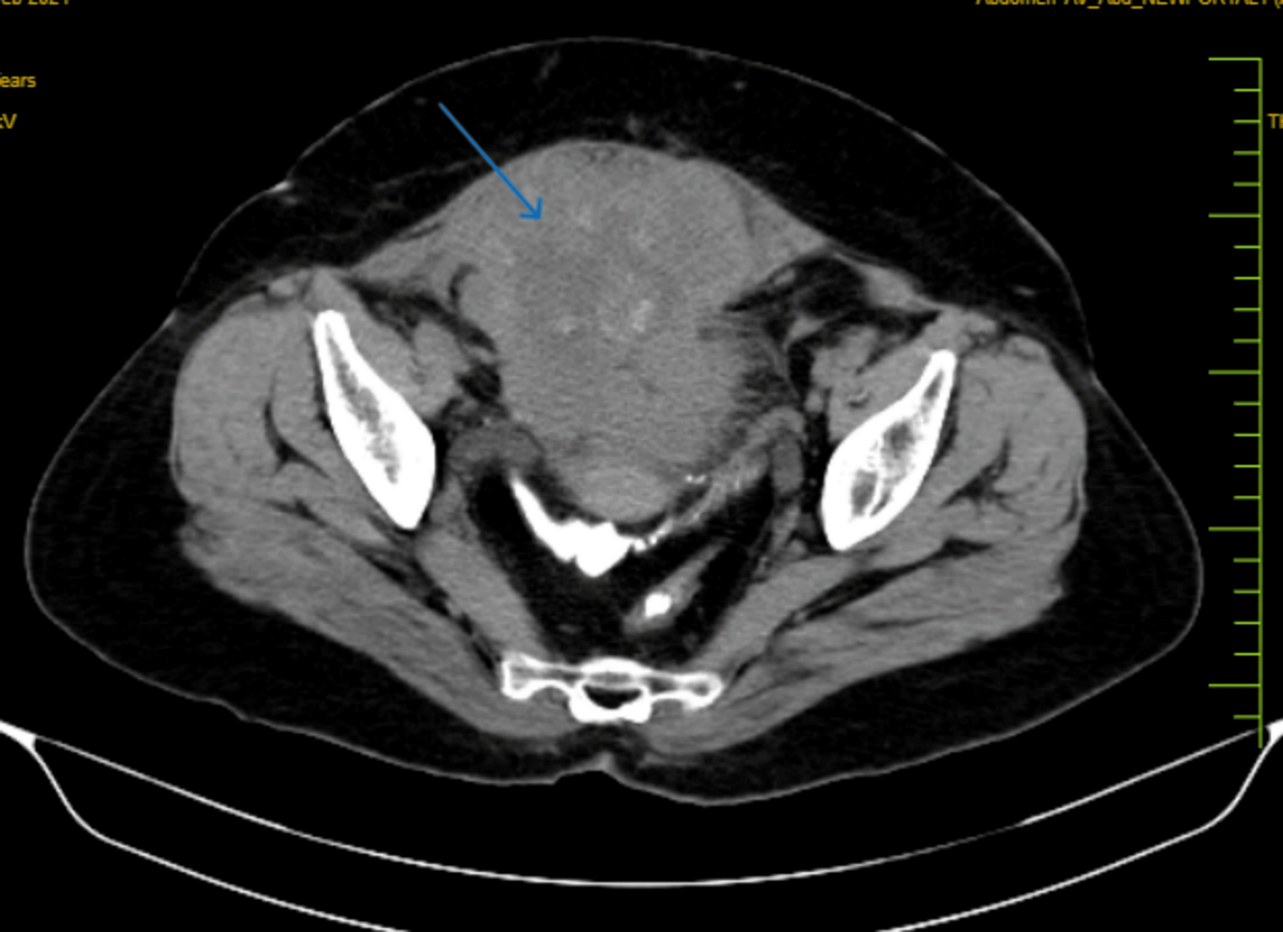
CT scan showing a heterogeneously enhancing lobulated soft tissue mass lesion (blue arrow) CT: computed tomography
